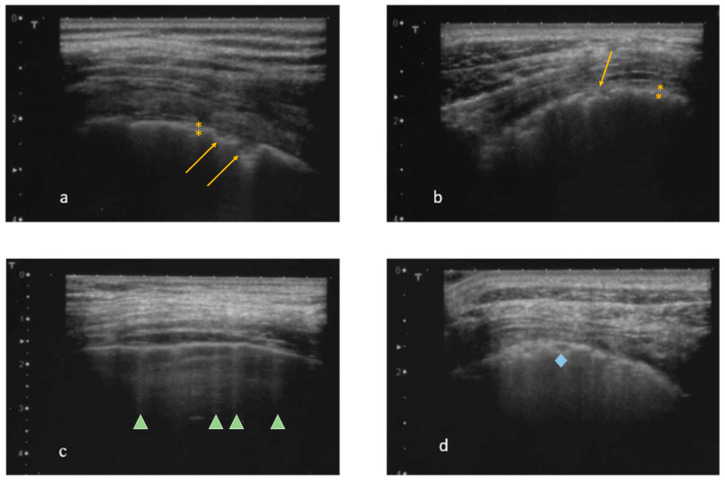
Echograms: signs detected by transthoracic lung ultrasound (linear probe between 7 and 10 MHz); (a) thickening (yellow asterisk) and irregularity (yellow arrow) of the pleural line; (b) thickening (yellow asterisk) and irregularity (yellow arrow) of the pleural line; (c) B-lines (green arrowheads); (d) subpleural cysts (blue rhombus).
